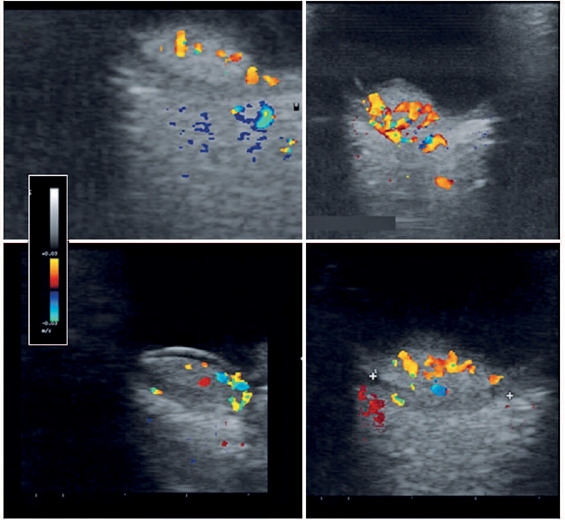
Color Doppler images (7.5–15-MHz linear transducer) from four patients
